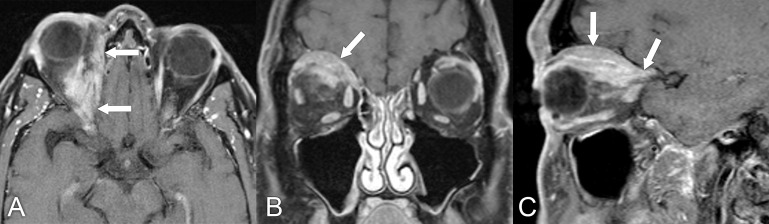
Magnetic resonance imaging in a 61-year-old man with slowly progressive right eye proptosis for the past year. (A–C) Axial, coronal, and sagittal, T1 postcontrast MRIs with fat suppression reveal a large infiltrating right superior orbital mass extending posteriorly to involve the orbital apex and superior orbital fissure, with further extension toward the right cavernous sinus (arrows). An anterior orbitotomy and biopsy of the lesion confirmed the diagnosis of marginal zone lymphoma. Systemic workup was otherwise negative for lymphoma.
